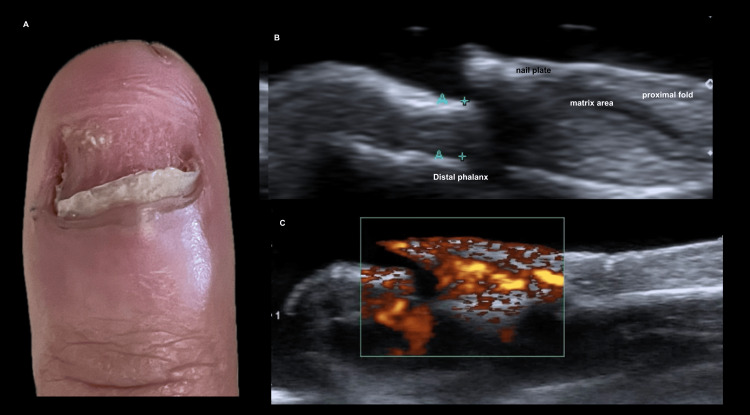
Nail plate destruction and chronic inflammation after acrylic nail removal. (A) Clinical image of a 22-year-old female patient showing near-complete loss of the nail plate following traumatic removal of acrylic nails. (B) Longitudinal grayscale ultrasound image demonstrating a residual hyperechoic segment of the nail plate with loss of normal ungual morphology. There is increased thickness of the proximal nail bed and matrix area (measured between blue calipers, A+). The hypoechoic nail bed also appears thickened, consistent with chronic inflammation. (C) Longitudinal power Doppler image showing intense vascularization, reflecting the degree of chronic inflammation.
